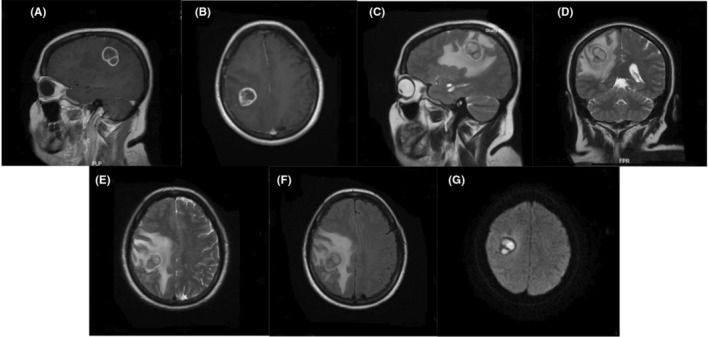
Mass with a calcified internal septum and ring enhancement in the right parietal lobe. (A) Sagittal T1‐Weighted, (B) Axial T1‐Weighted, (C) Sagittal T2‐Weighted, (D) Coronal T2‐Weighted, (E) Axial T2‐Weighted, (F) Axial T2‐weighted‐Fluid‐Attenuated Inversion Recovery (FLAIR), (G) Diffusion‐weighted MRI (DWI)
